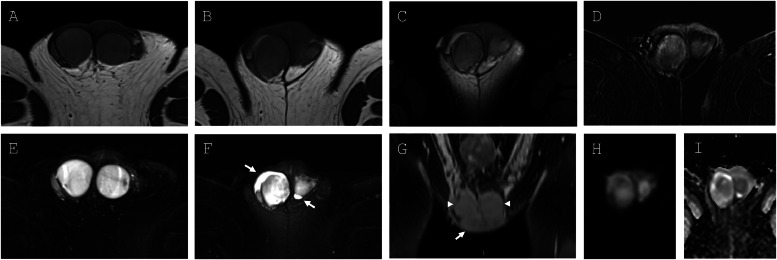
(A) T1-weighted axial images of the testes and (B) right SNT, demonstrating homogenous, intermediate signal intensity. (C) T1-weighted axial image of right SNT shows no signal dropout with fat saturation, confirming absence of intralesional fat. (D) T1-weighted postcontrast axial image of right SNT shows heterogeneous enhancement, suggestive of previous vascular or infectious insult. (E) T2-weighed axial fat saturated images of the testes and (F) right SNT, with similarly homogenous T2 hyperintensity. Bilateral hydroceles present (arrows). (G) T1-weighted postcontrast coronal image of bilateral testes (arrowheads) and right SNT (arrow). (H and I) Diffusion-weighted images and corresponding ADC map demonstrating low-level diffusion restriction within the right SNT.
